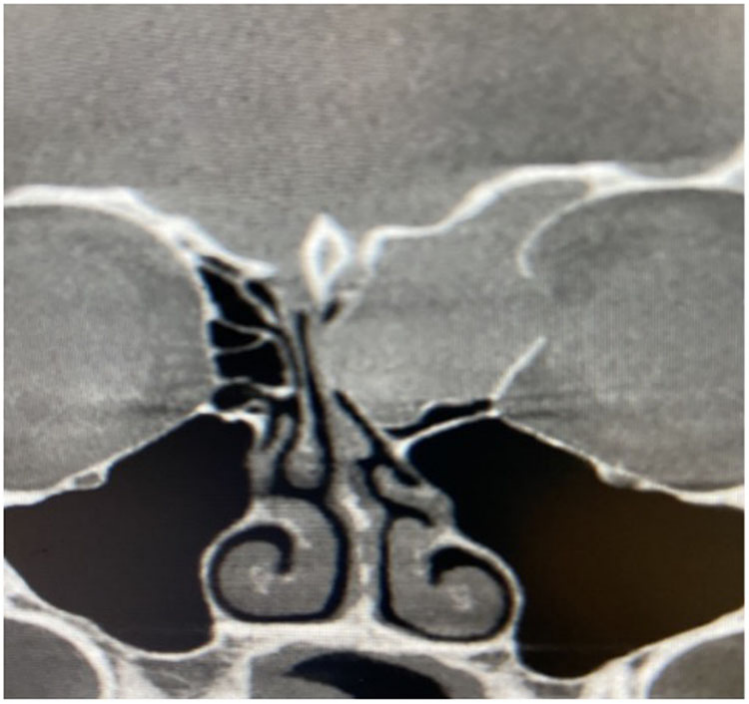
Computed tomography sinus. It shows frontal ethmoid mucocele destroying the left lamina papyracea. Note intact frontal sinus.
